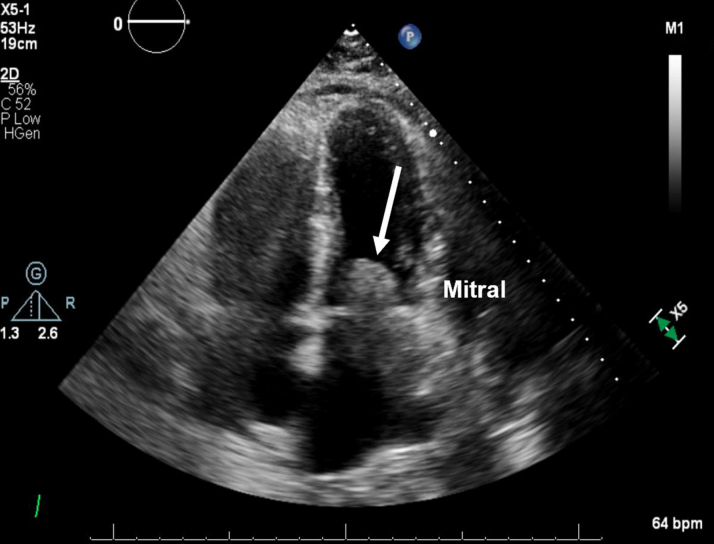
Transthoracic Echocardiography at Day 20 Showing a Rapidly Growing Obstructive Mass Crossing the Mitral Valve From the Left Atrium Into the Left Ventricle Cavity The arrow points to the obstructive mass.
